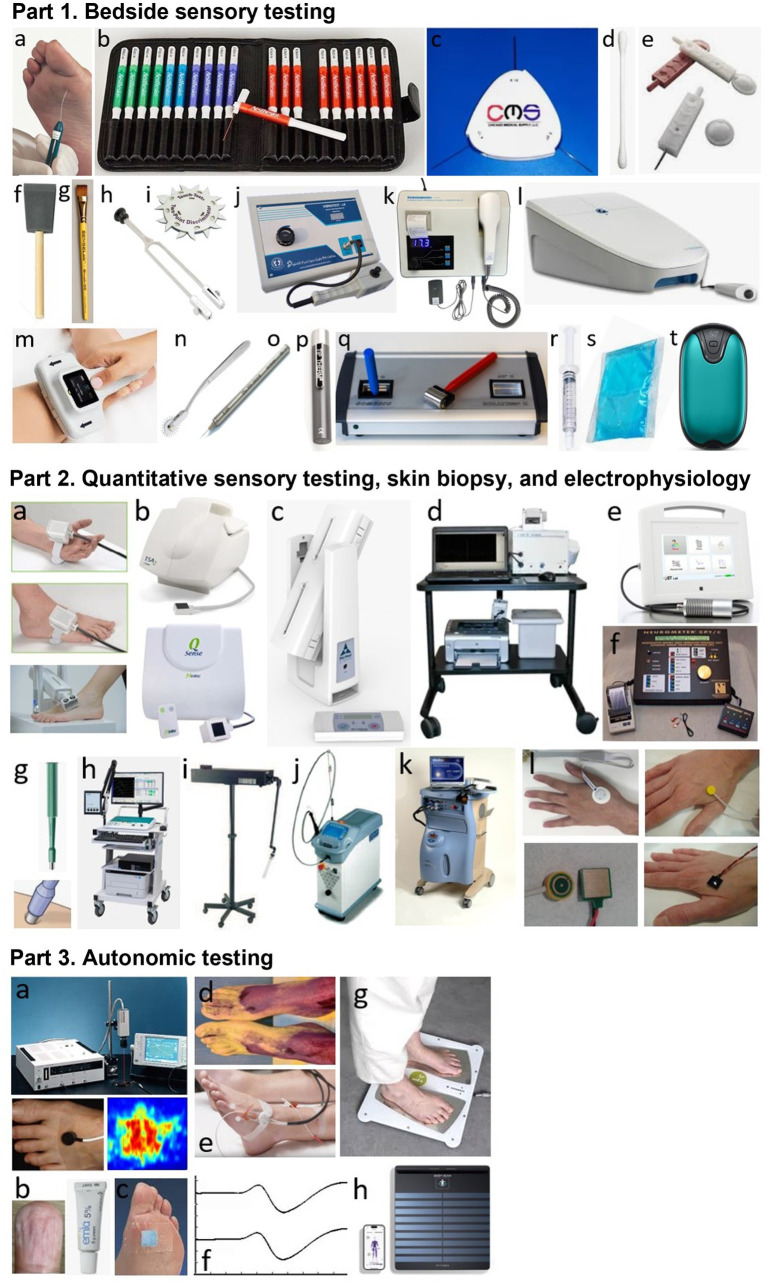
Part 1. Bedside sensory testing. a: 10-g monofilament, b: von Frey/Semmes-Weinstein monofilaments testing kit, c: 1/10/75-g nylon filament wheel, d: Q-tip, e: sharp and round tips of Neurotip^®^, f: foam brush, g: calibrated hair brush, h: 128-Hz (Rydel-Seiffer) tuning fork, i: two-point discriminator wheel, j: Vibrometer^®^, k: Biothesiometer^®^, l: VibroSense^®^, m: DPNCheck®, n: Wartenberg wheel, o: Pin prick^®^ stimulator, p: Tip Therm^®^, q: Rolltemp^®^, r: filled syringe, s: cooling pack, t: digital hand warmer. Part 2. Quantitative sensory testing, skin biopsy, and electrophysiology. a: thermodes, b: TSA2® and Q-Sense^®^, c: NerveCheck^®^, d: Case IV^®^, e: QST. Lab^®^, f: Neurometer^®^, g: disposable skin biopsy punch, h; machine for performing nerve conduction study or evoked potentials, i: CO2 laser, j: Nd: YAP laser, k; contact-heat evoked potentials, l: different electrodes for performing intraepidermal evoked potentials. Part 3. Autonomic testing (at foot level). a: Laser doppler flowmetry or imaging, b: EMLA test, c: Neuropad^®^, d: thermoregulatory sweat test, e: QSART^®^, f: sympathetic skin response, g: Sudoscan^®^, h: Body Scan^®^.
